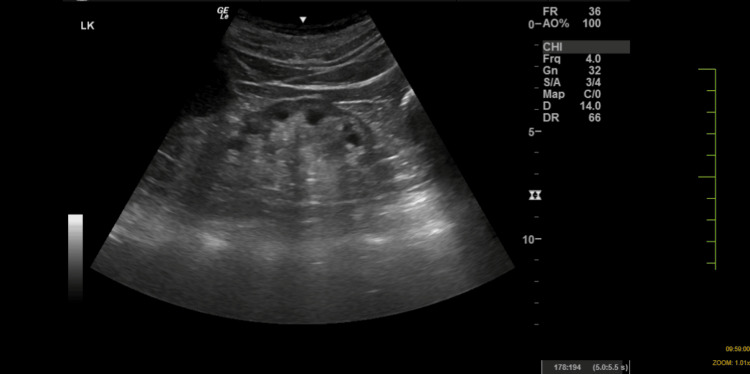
Ultrasound abdomen showing the left kidney The left kidney appears small in size with poor differentiation of the corticomedullary junction.
